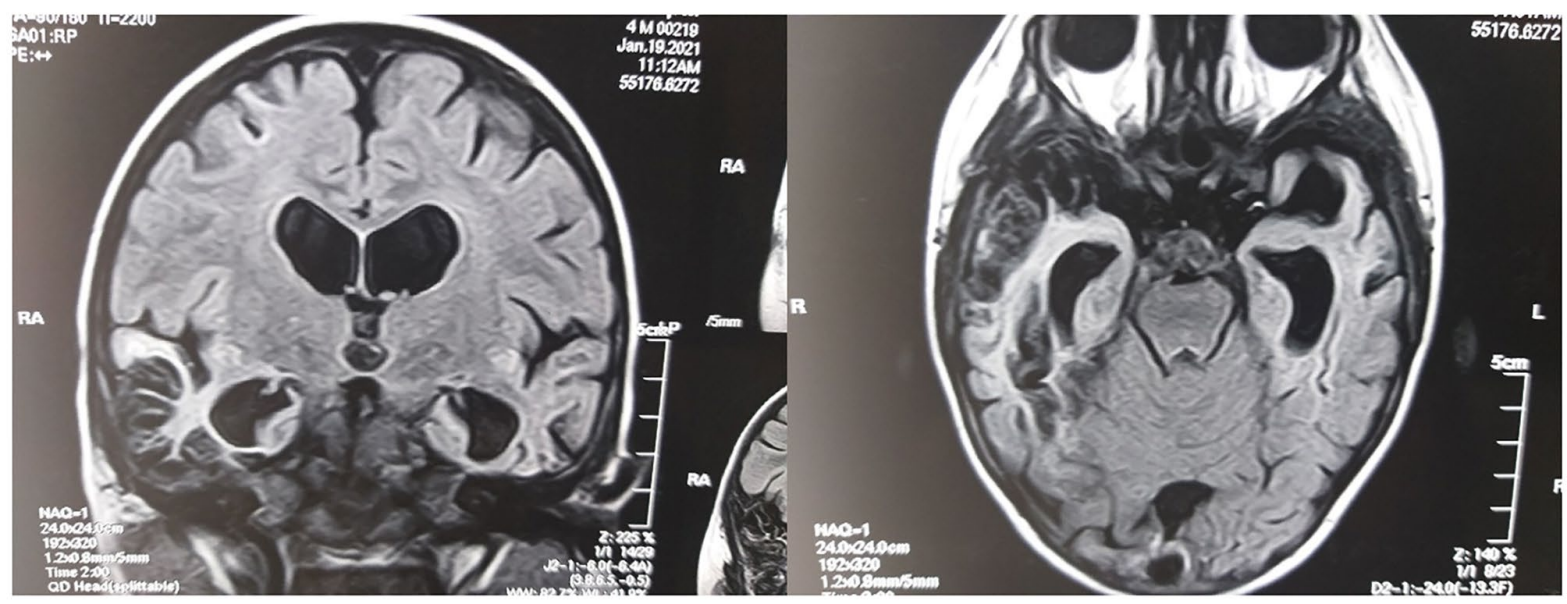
The MRI of the brain in FLAIR mode in coronary (left) and axial (right) projections in a child with ASD associated with GFCD. There are signs of bilateral atrophy and cystic gliosis changes in the temporal lobes of the cerebral hemispheres after bilateral temporal necrotic-hemorrhagic HSV-1-etiology encephalitis (the authors’ observation)
